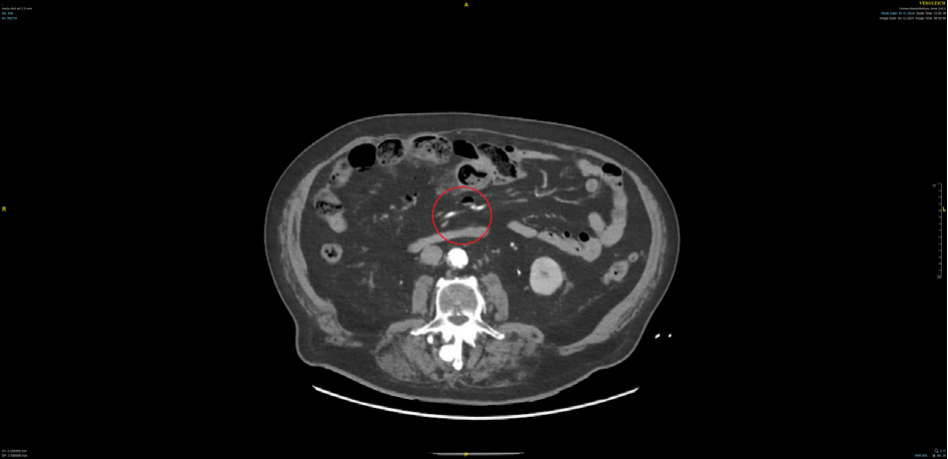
Axial view of an abdominal angiographic computed tomography scan. Red circles mark gases in the venous system.
